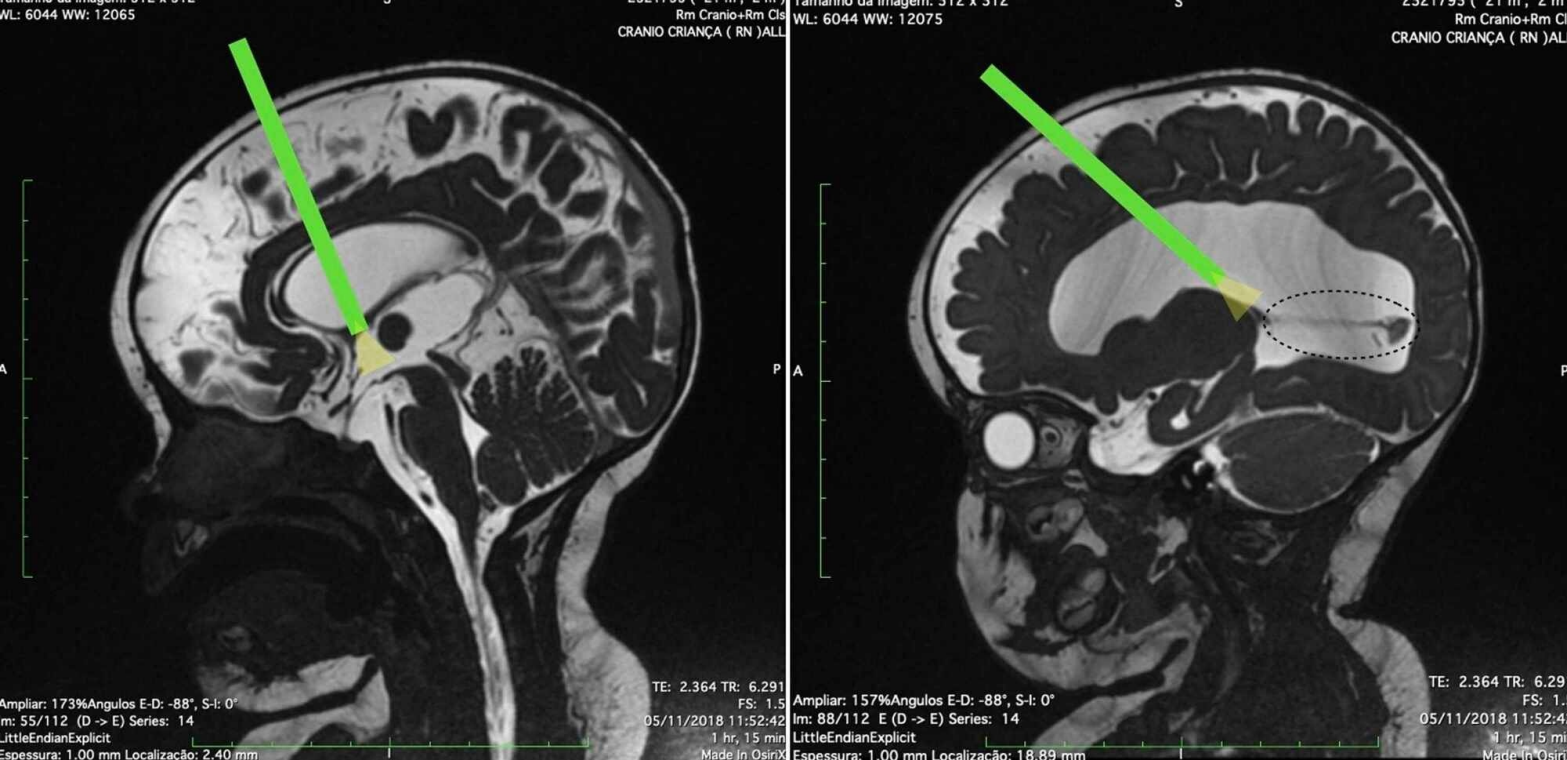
Planning of endoscopic third ventriculostomy (ETV) and choroid plexus cauterization with selective resection of the glomus in a patient with a type III variant. The sagittal view on T2-weighted magnetic resonance imaging (MRI) depicted the estimated angle of the endoscope during ETV (left). Through the same entry point, MRI allowed us to estimate the angle needed to reach the fixation of the glomus on the pulvinar nuclei of the thalamus (right). The green rectangle represents the endoscope, and the yellow triangle represents the illumination.
